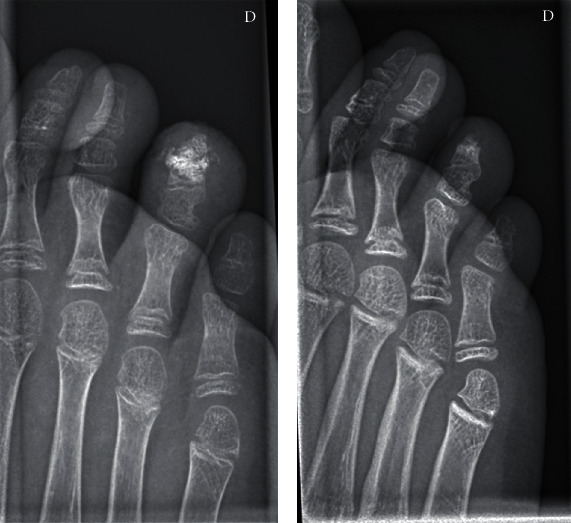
(a) X-ray demonstrating the lyophilized bone graft three week after the intervention. (b) X-ray demonstrating the complete incorporation and remodeling of the graft one year after the intervention.
